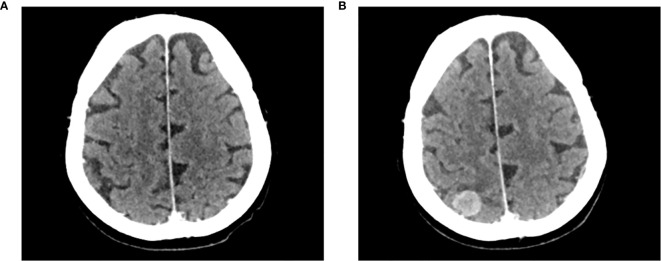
Development of an asymptomatic brain metastasis in patient case 1. Computed tomography brain scans of patient case 1 prior to initiating treatment with avelumab (A) on August 16, 2017, and (B) on October 2, 2017.
